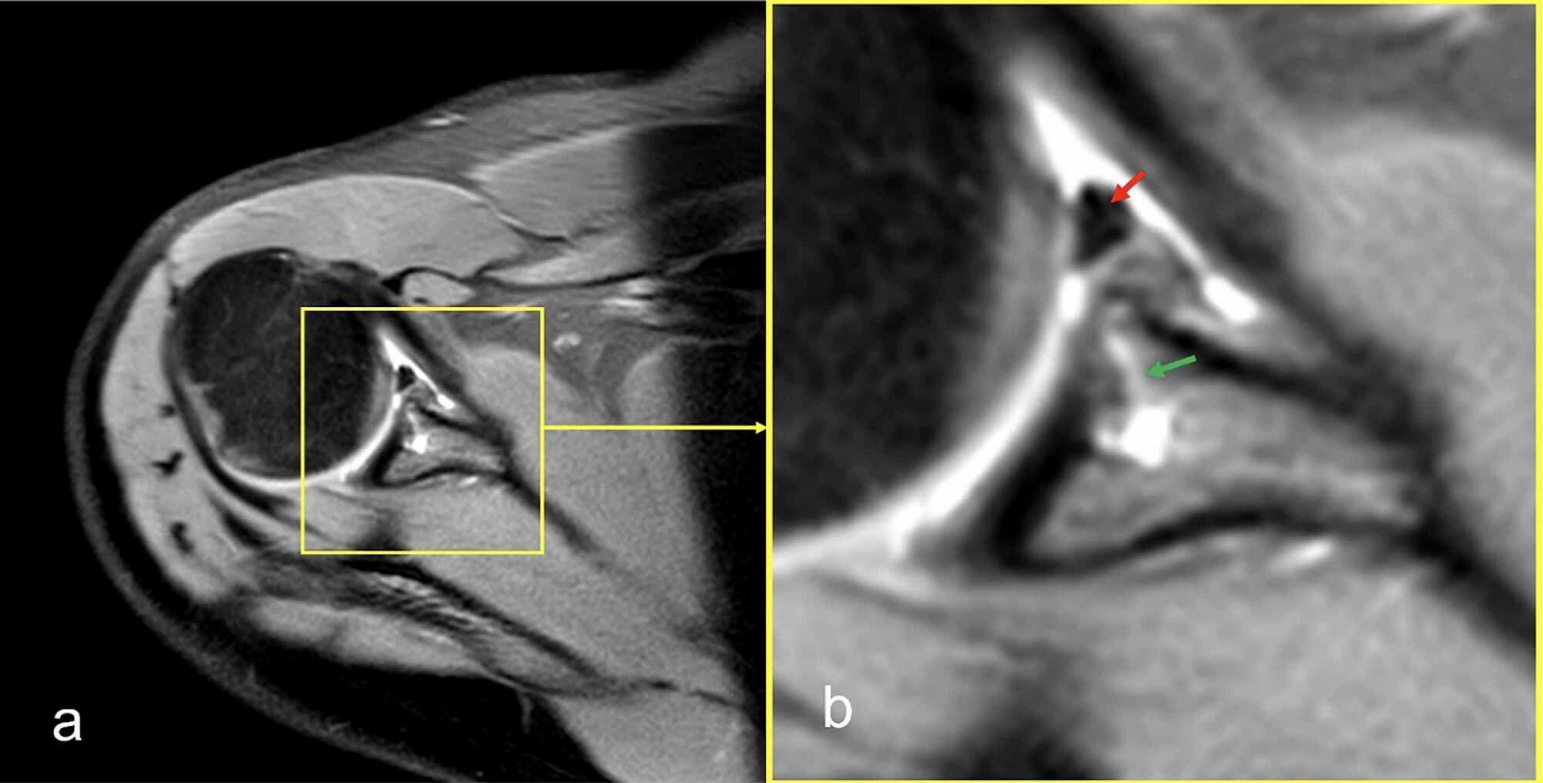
Postoperative MRI images of case 2 (a) An axial image showing totally fixed LHBT to glenoid; (b) a magnification view of MRI showing the LHBT in the glenoid (red arrow) and anchor (green arrow). LHBT: long head of the biceps tendon.
